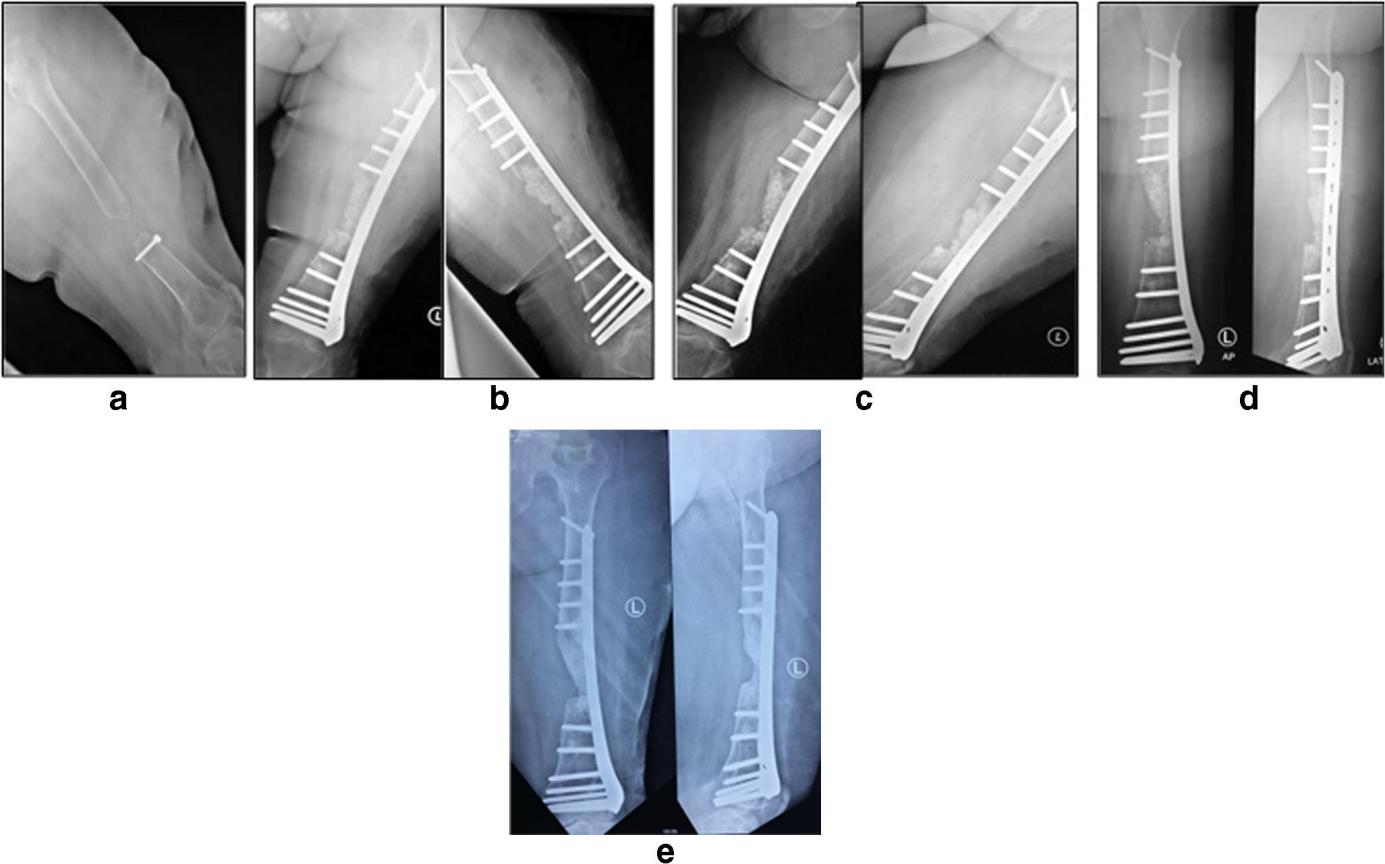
A 40-year-old female with 5 years of 6-cm bone defect of the femur. a Pre-operative radiography. b Post-operative radiography. c Six-month post-operative radiography. d Twelve-month post-operative radiography. e Four-year post-operative radiography
